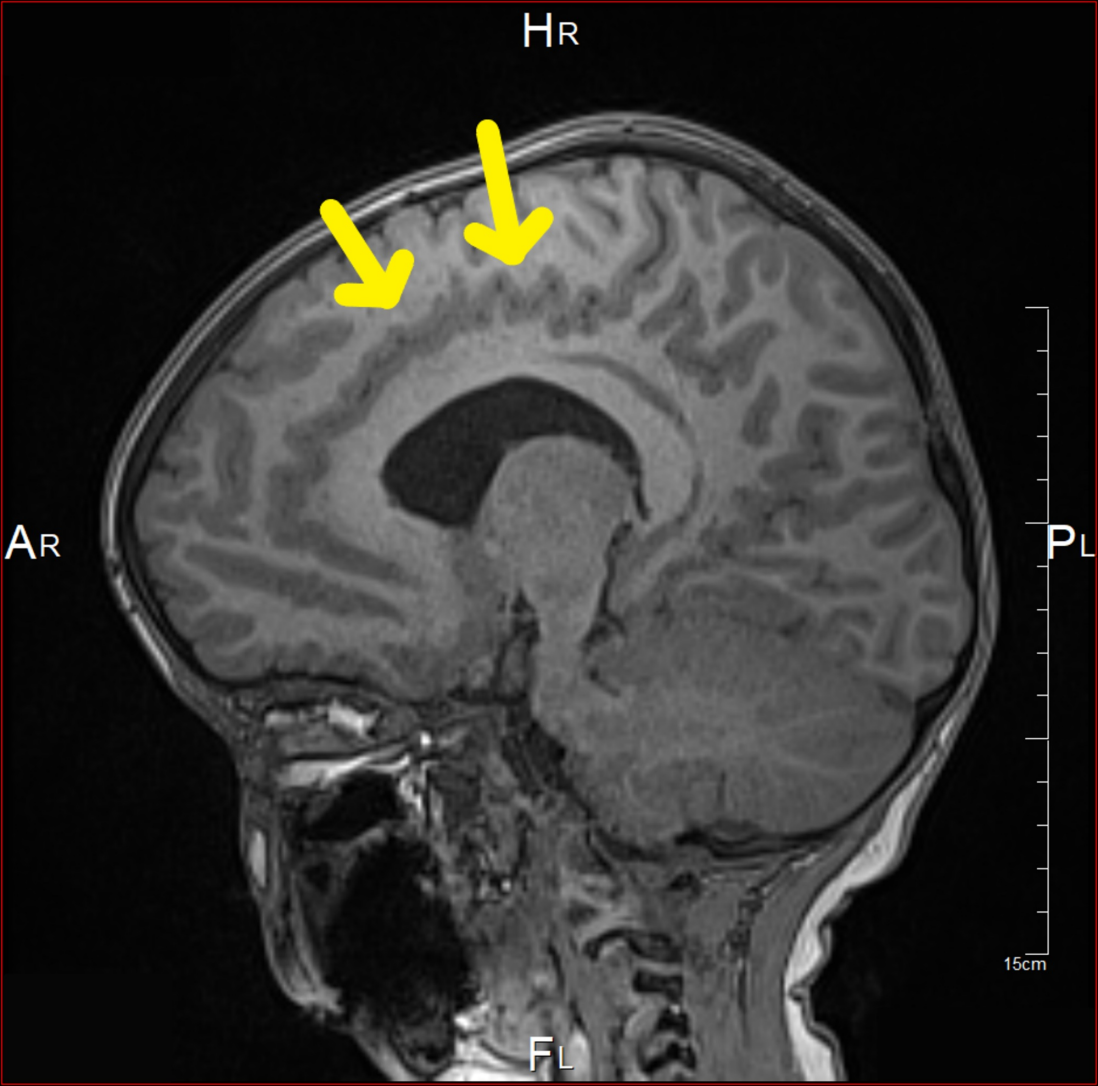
Brain MRI showing bifrontal and perisylvian polymicrogyria
